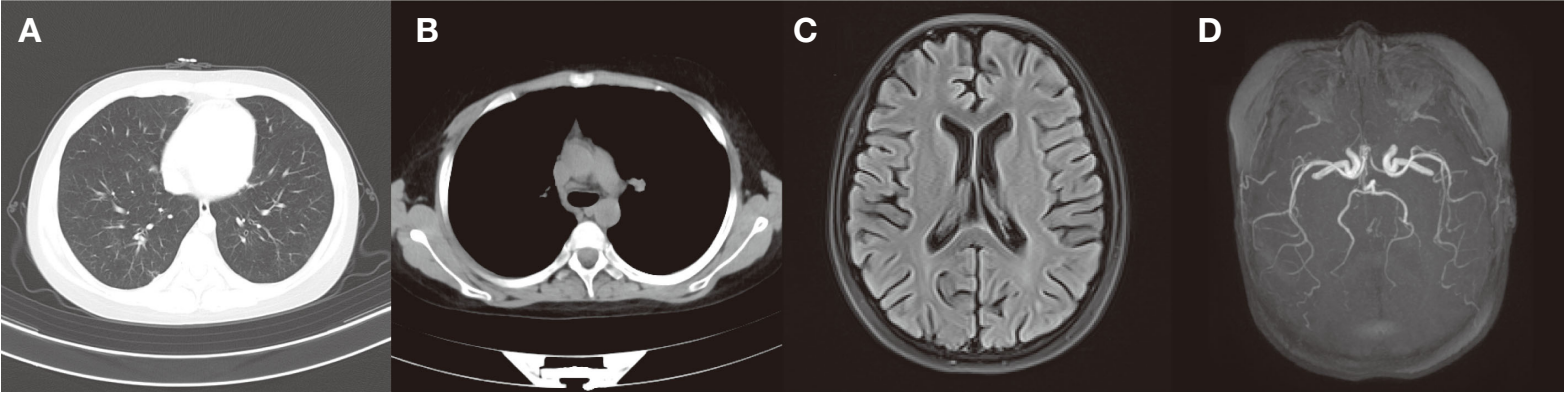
(A, B) After treatment, the chest CT scans revealed significant improvement in lung inflammation and no obvious enlarged lymph nodes were seen in the mediastinum and bilateral hilar. (C) After treatment, the cranial MRI showed a reduction in the abnormal signal intensity in the bilateral cerebral cortex compared to the previous scan. (D) After treatment, there were no markedly abnormal findings in cranial MRA.
